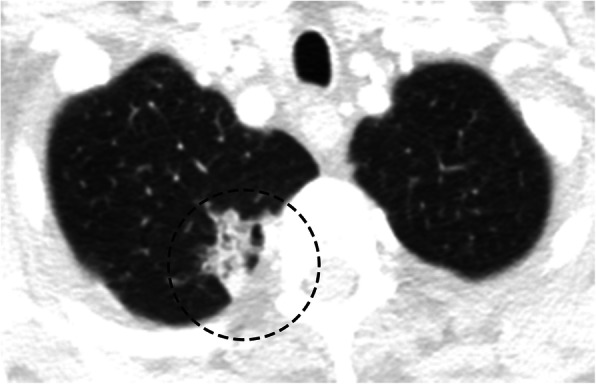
Adenocarcinoma of the lung. Axial image of a CT chest demonstrating an irregular shaped ground glass semisolid lesion in the apex of the right lung (dashed circle) in the setting of biopsy proven invasive adenocarcinoma
